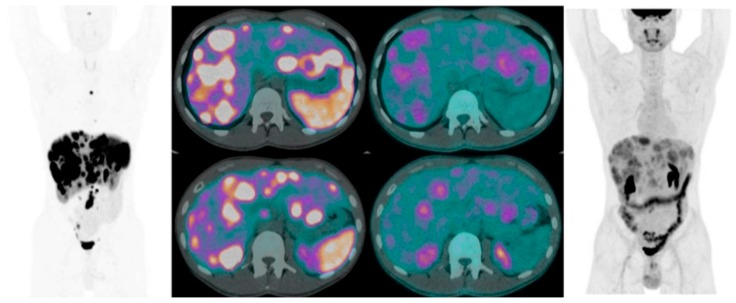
Twenty-four-year-old male patient with newly diagnosed ileal neuroendocrine neoplasia (NEN) with liver, lymph node and bone metastases. Combined imaging with [^68^Ga]Ga-DOTA- NaI^3^-Octreotide (NOC) PET/CT (left) and [^18^F]FDG PET/CT (right) show the heterogeneity of the disease.
